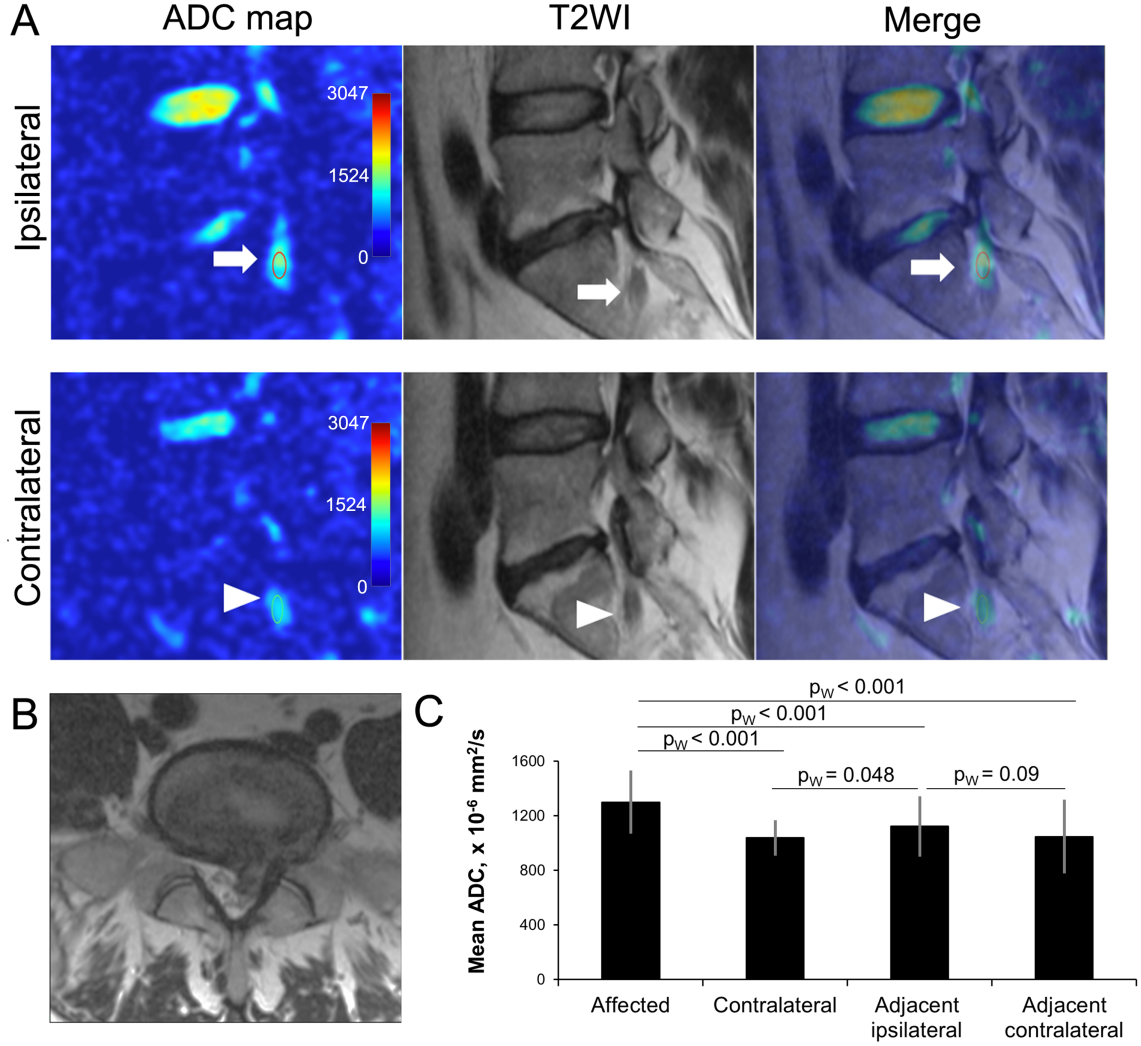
Assessment of nerve root ADC. (A) ADC maps, T2-WI and merged images of left (ipsilateral) and right (contralateral) parasaggital lumbar scans of 38 y.o. male patient with right sided L5-S1 disk herniation. Regions of interest (15 mm^2^) were selected over the ipsilateral (arrows) contralateral (arrowheads) S1 nerve roots. (B) Axial T2-WI showing disk herniation. (C) Comparison of ADC values from the affected, contralateral, adjacent ipsilateral (S1 or L5), and adjacent contralateral nerve roots. Data calculated from 19 patients with symptomatic disk herniations. *—p < .01. ADC, apparent diffusion coefficient; T2-WI, T2-weighted image.
